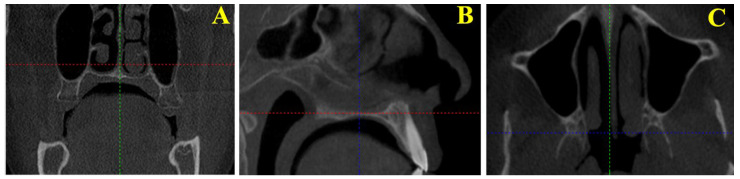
(A) Positioning of the sagittal reference line in the coronal plane by passing through the midpoint of the anterior nasal spine on cone-beam computed tomography (CBCT) images. (B) Identification of reference points in the sagittal plane for axial evaluation on CBCT images. (C) Selection of the axial slice that best visualizes the pterygomaxillary region between the predetermined reference points on CBCT images.
